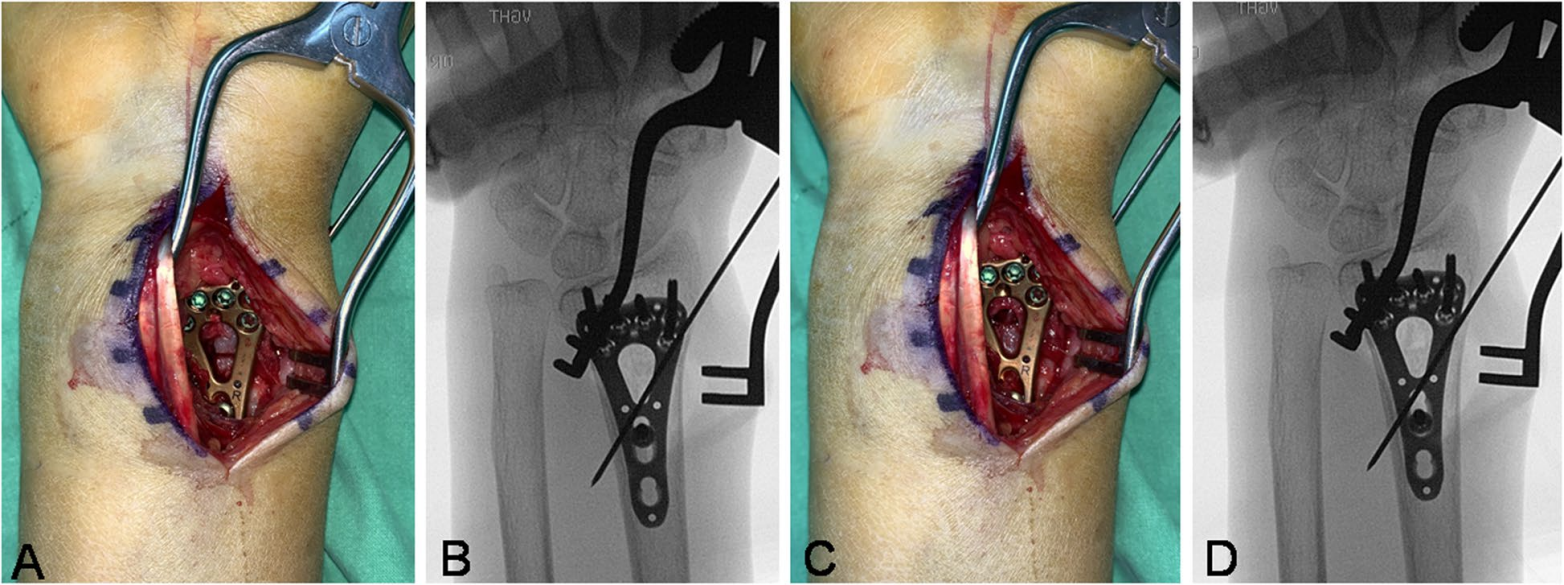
Demonstrating the indirect ulnar shortening after radial distraction via radiographs and photographs. A Before distraction, the volar cortex was contact, B Before distraction, the ulna-plus morphology was found in the intraoperative radiograph after reduction, C After distraction, the gap of the volar cortex was found, D After distraction, the positive ulnar variance became smaller than before distraction
